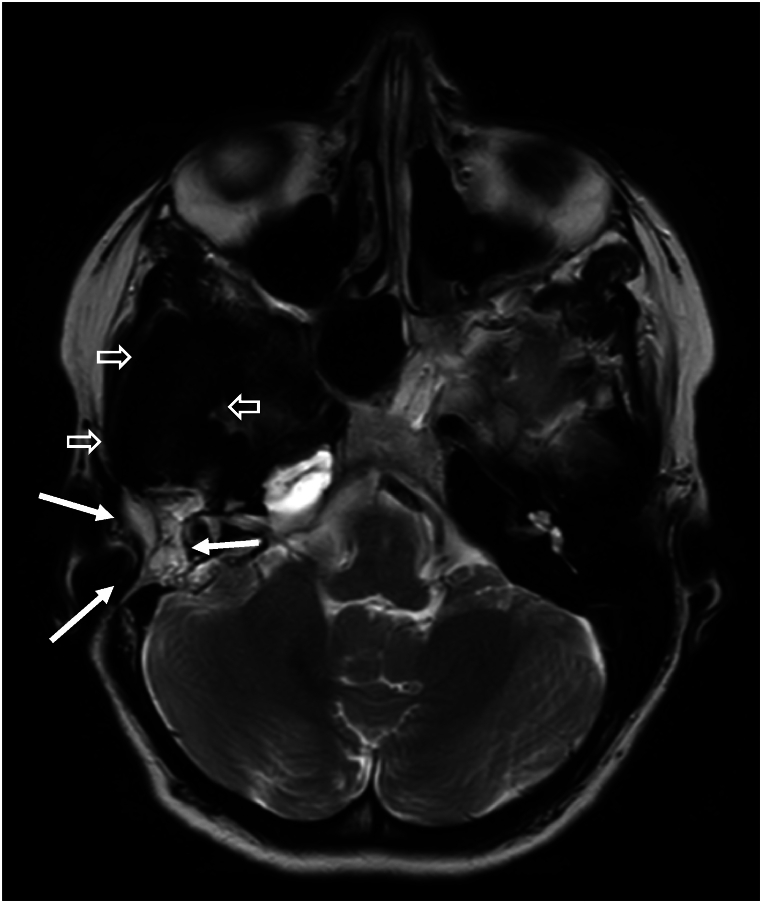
Axial T2-weighted imaging. Hyperintense (fatty) tissue visible after subtotal petrosectomy (white arrows). Persistent hyperostosis of the sphenoid bone anterior of the petrosectomy (hypo-intense signal, open arrows).
